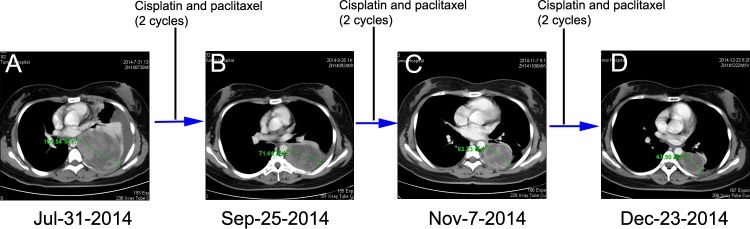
Chest CT scans documenting responses to cisplatin and paclitaxel (CP) chemotherapy A. Chest CT at the initial presentation reveals showing a pace-occupying mass in the left lung (July 31, 2014). Chest CT scan was taken after every two cycles of CP B. and C. After six cycles of CP treatment, CT image showing a PR (Dec 23, 2014) by RECIST D..
